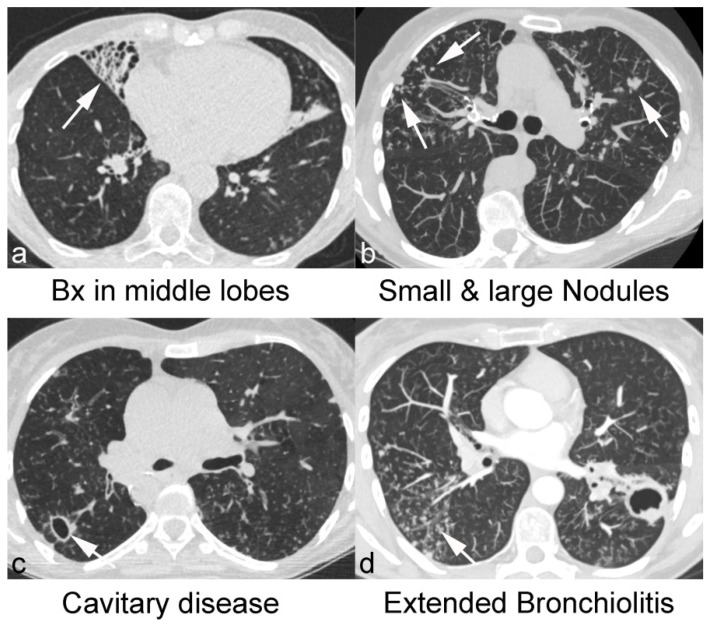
CT findings in patients with NTM-PD.
